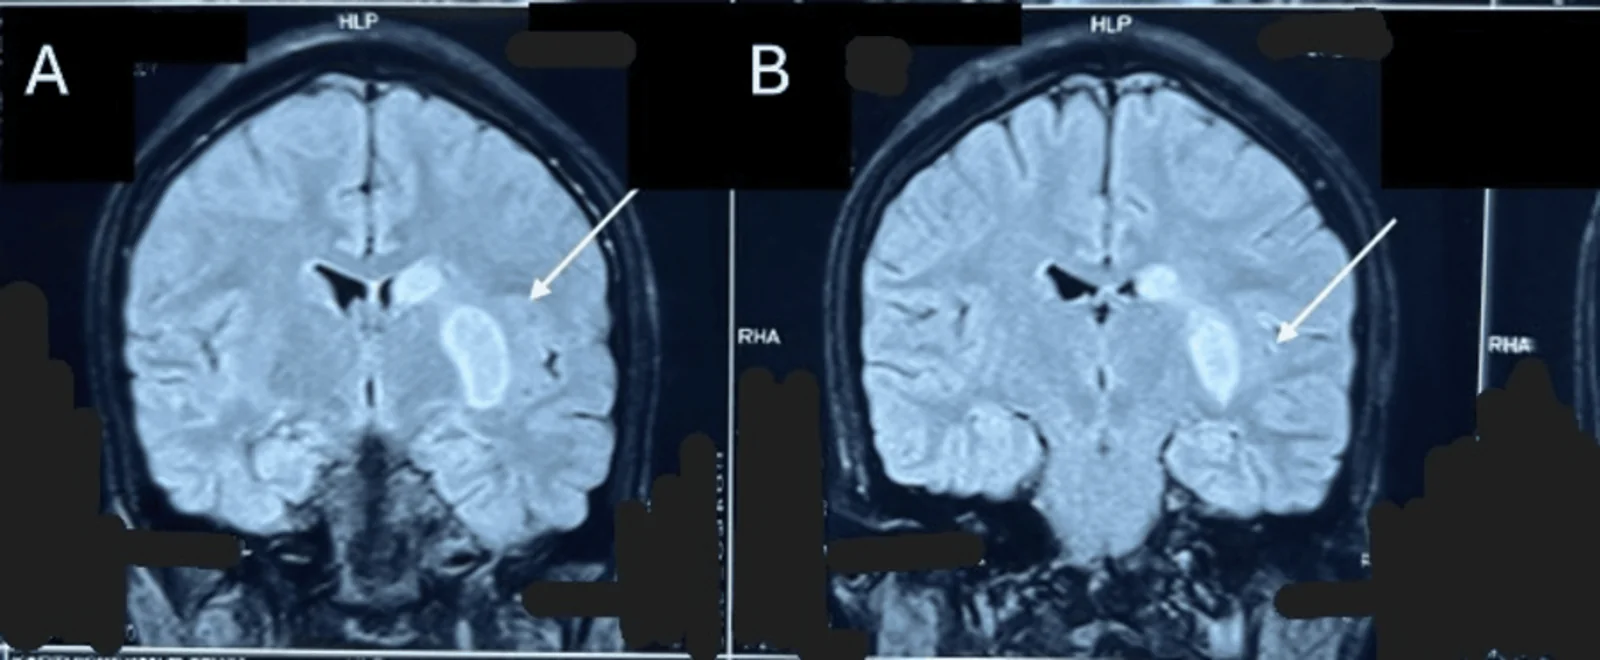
MRI brain showing large area of diffusion restriction within blooming within noted in left ganglio-capsular region suggestive of acute infarcts with hemorrhagic changes.
